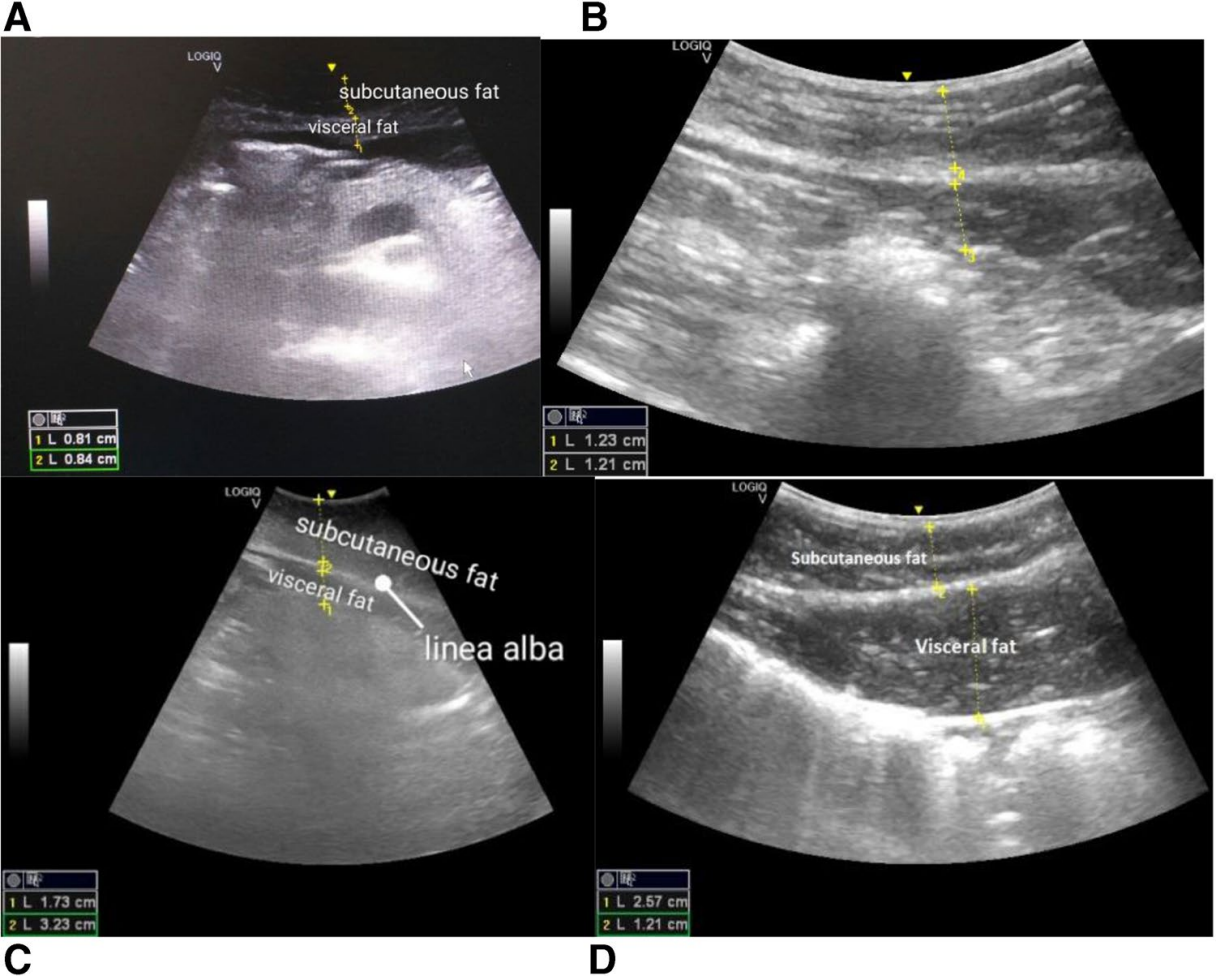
Ultrasound imaging as the diagnostic tool to discriminates between A abnormally low and B excessive versus C normal abdominal and visceral fat distribution and D SAT patterns in visceral fat redistribution; evident gender difference is well respected by gender-specific patterns of fat distribution, namely for males (B and D) and females (A and C). Notably, specific movement patterns at breathing further contribute to the correctness of the fat tissue measurement in abdominal cavity. The scanning was performed in the sagittal plane along the linea alba; the figure is adapted from [4]
